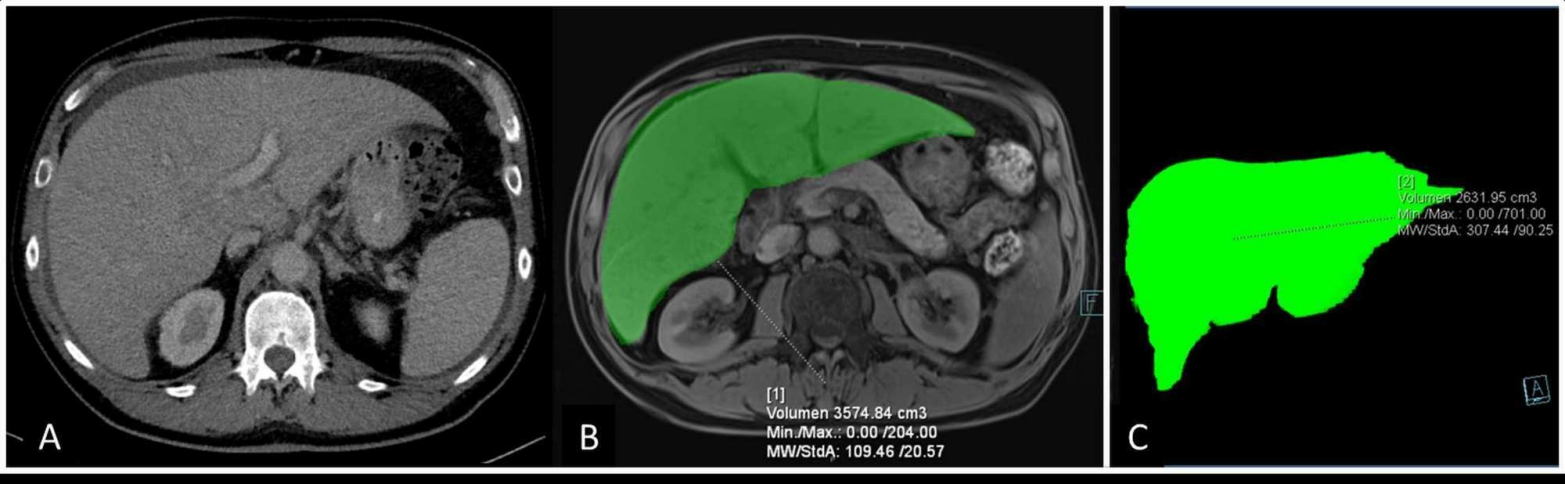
Findings on cross sectional imaging on presentation and during the follow-up Computed tomography (CT) scan on presentation showing a clearly enlarged liver (Panel A). Magnetic resonance imaging (MRI) in February 2016 showed a stable hepatomegaly (Panel B), which improved substantially on VGPR one year later (volumetric reconstruction of MRI liver images; Panel C). VGPR: very good partial response.
